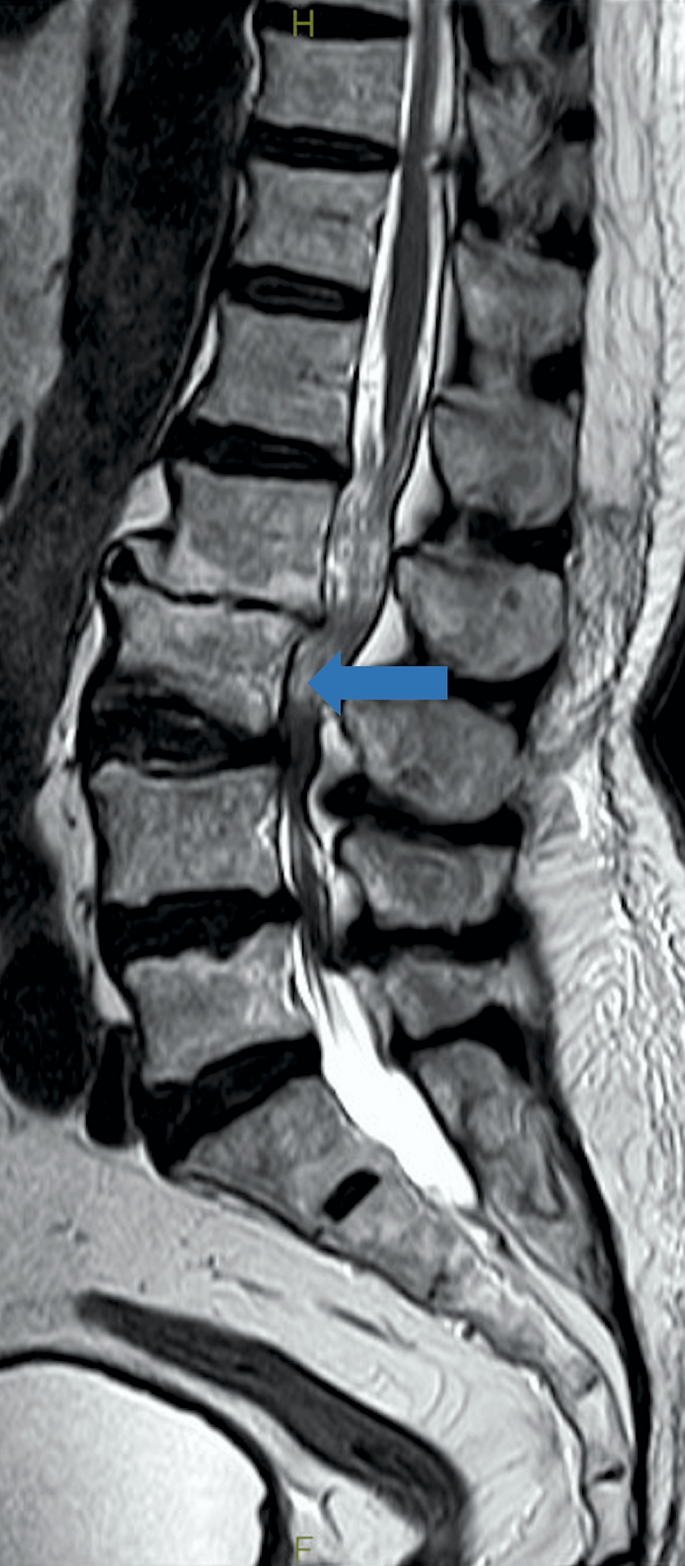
Magnetic resonance imaging of the lumbar spine on arrival revealed an L3 fracture (blue arrow).
